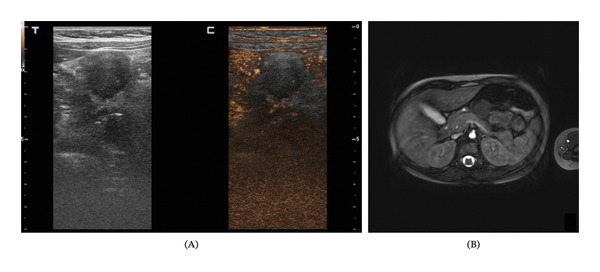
(A) 21‐mm, well‐defined, rounded hypoechoic lesion with heterogeneous internal echotexture is visualized within the perigastric fat on ultrasound. (B) MRN imaging. In the epigastric adipose tissue, at the interface between the stomach, pancreas, and proximal jejunal loops, a nodular lesion measuring approximately 22 × 23 × 24 mm is identified. The lesion appears distinctly hypointense on T2‐weighted sequences.
